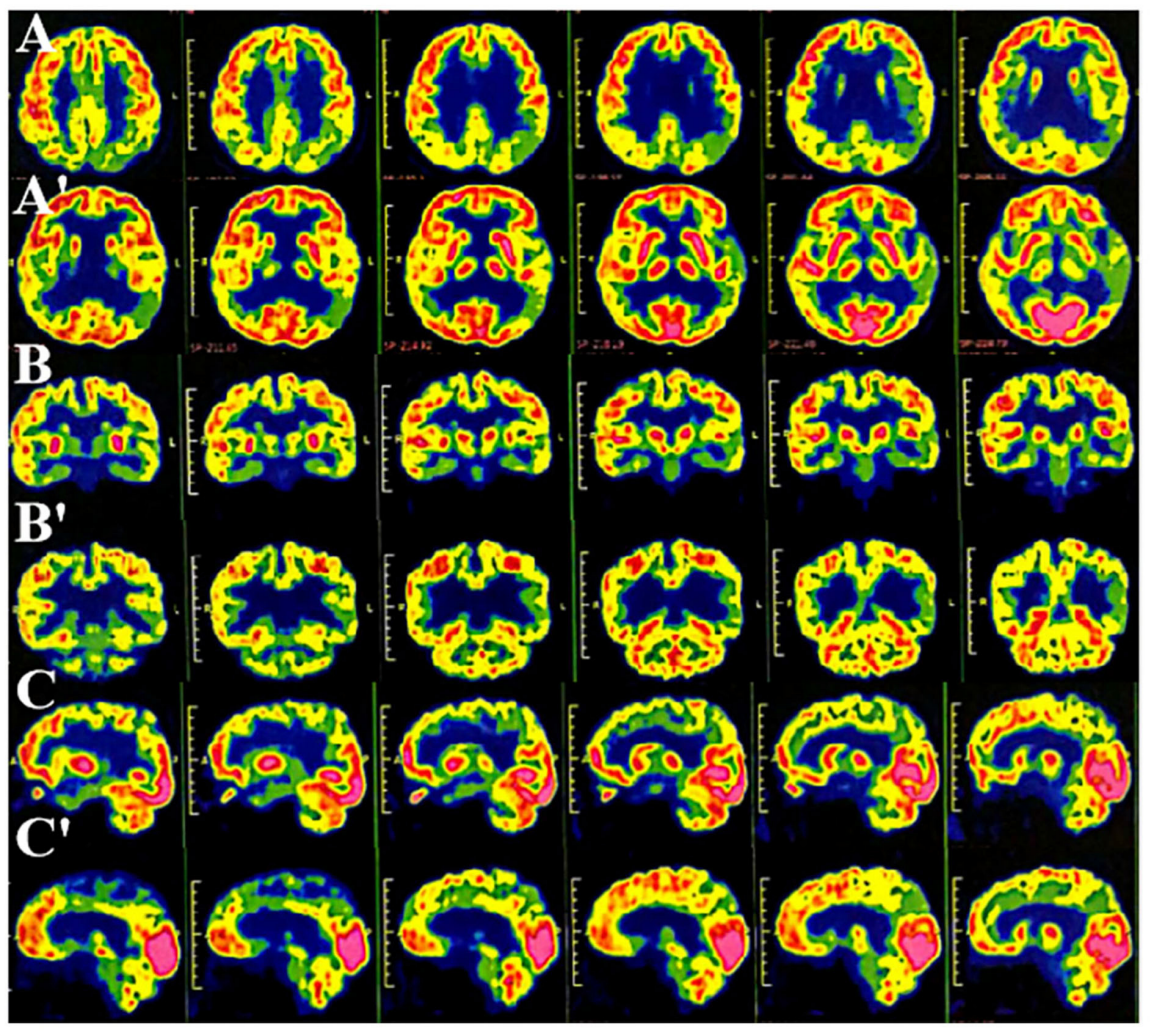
Multimode molecular imaging of 18-fluoro-2-deoxy-D-glucose positron emission tomography (^18^F-FDG PET-CT) showed reduced multiple hypometabolism in the bilateral parietal and temporoparietal junction, bilateral temporal lobes (left), and posterior cingulate gyrus (AA': cross-sectional sequence; BB': coronal image; CC': sagittal image).
